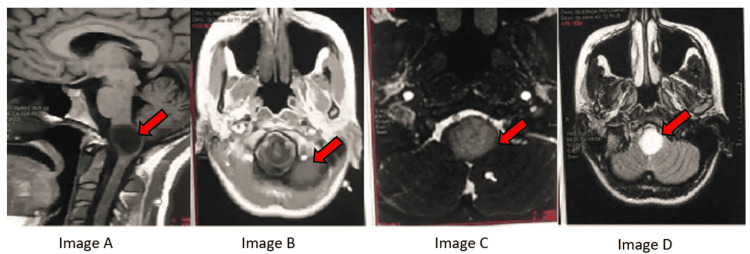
(A) Sagittal T1: hypointense cystic brain stem mass (red arrow). (B) Axial T1+C: nodular enhancement (red arrow). (C) Axial T2 FLAIR: iso/hyperintense (red arrow). (D) Axial T2: hyperintense (red arrow). FLAIR: fluid-attenuated inversion recovery
